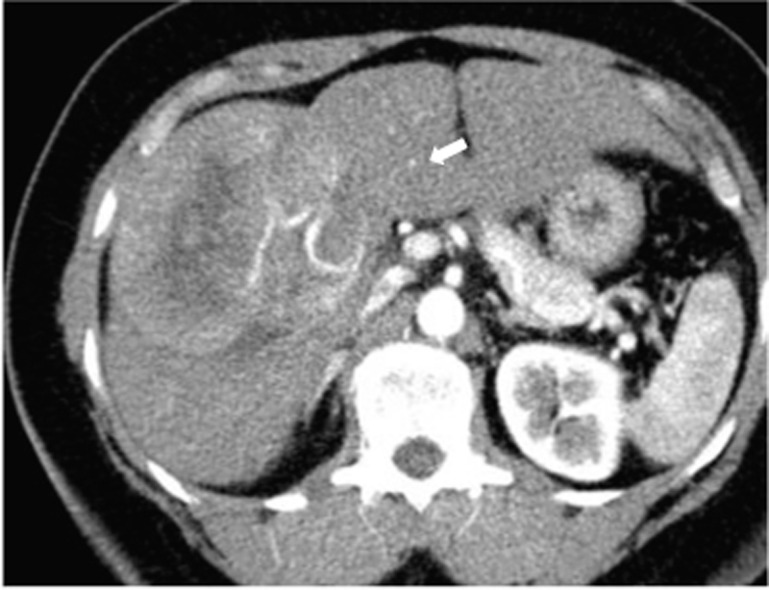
Triphasic CT (Arterial Phase): Enhanced tumor (arrow) with hypodense center and fatty liver.
